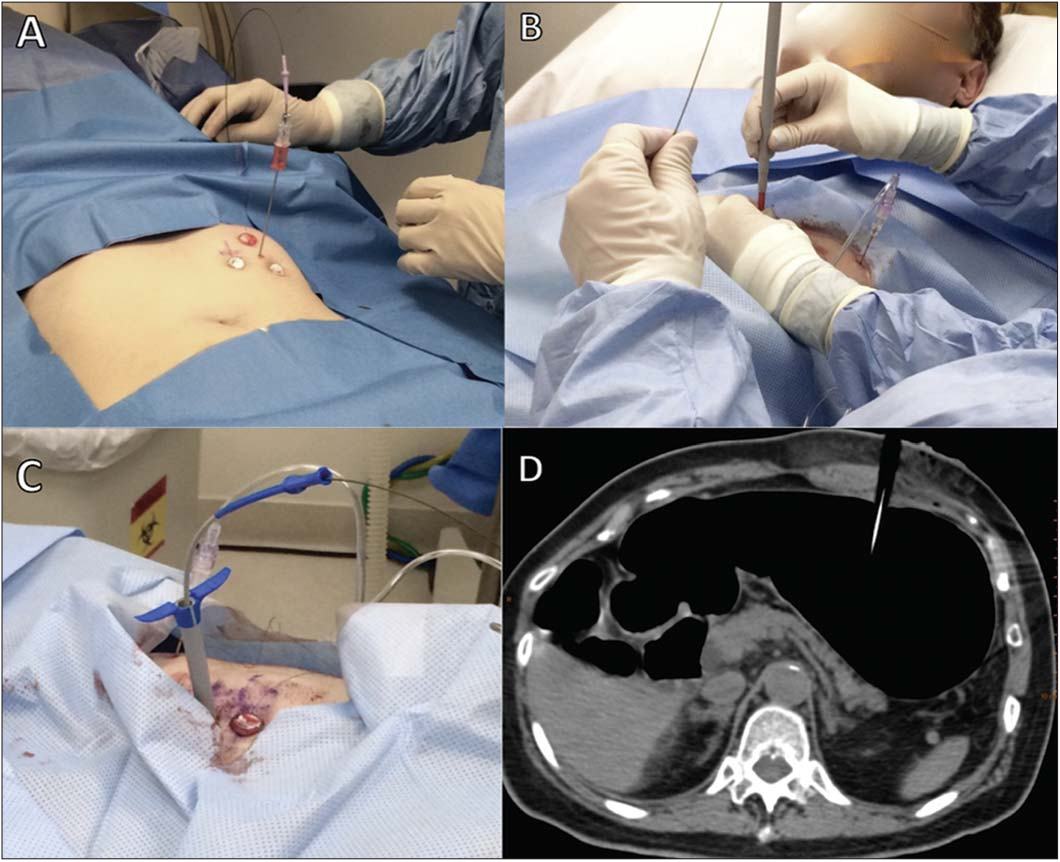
Passage of the guidewire and dilators for CT-guided
